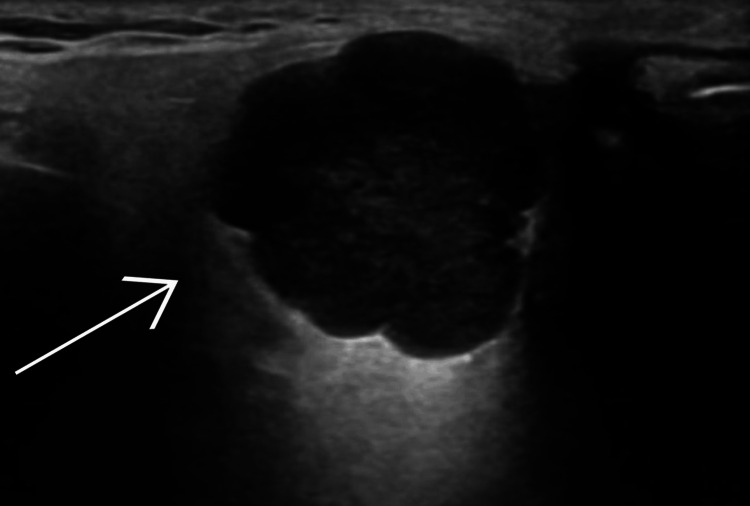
Ultrasonography of superficial swelling of the left parotid gland showing features suggestive of a pleomorphic adenoma marked by a white arrow.
